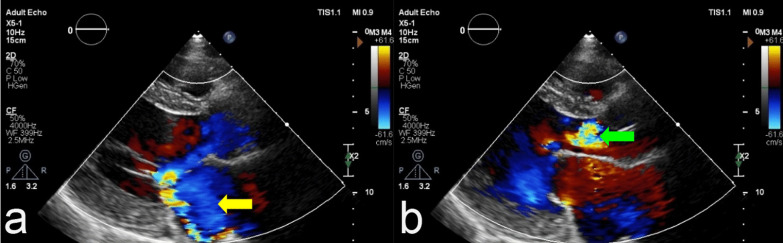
Echocardiogram showed left atrial and ventricular dilatation with severe a mitral valve regurgitation (yellow arrow) and b aortic valve regurgitation (green arrow)
